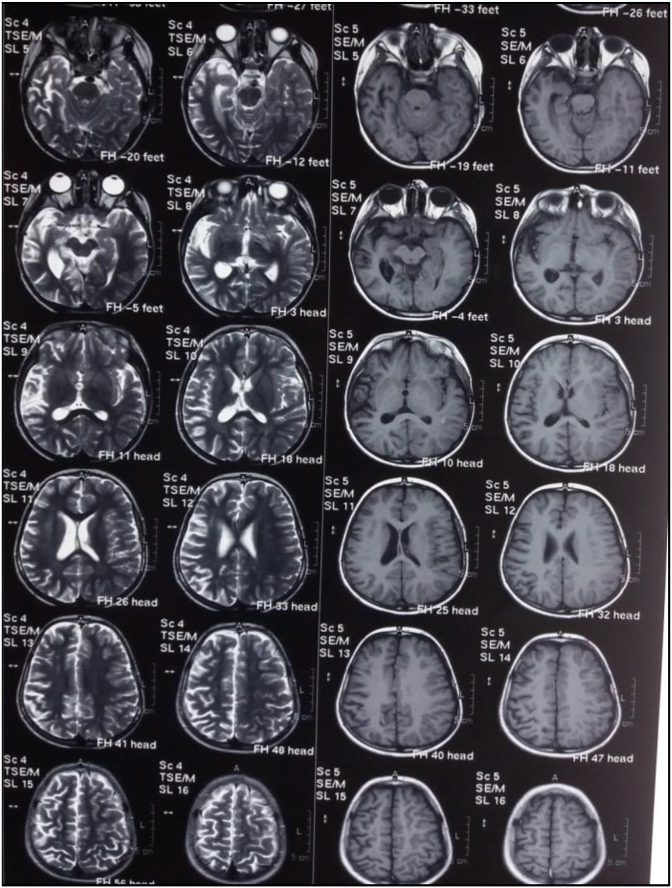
MRI T1 (B) and T2 (A)(Axial) show atrophy in right partial and temporal lobes.
